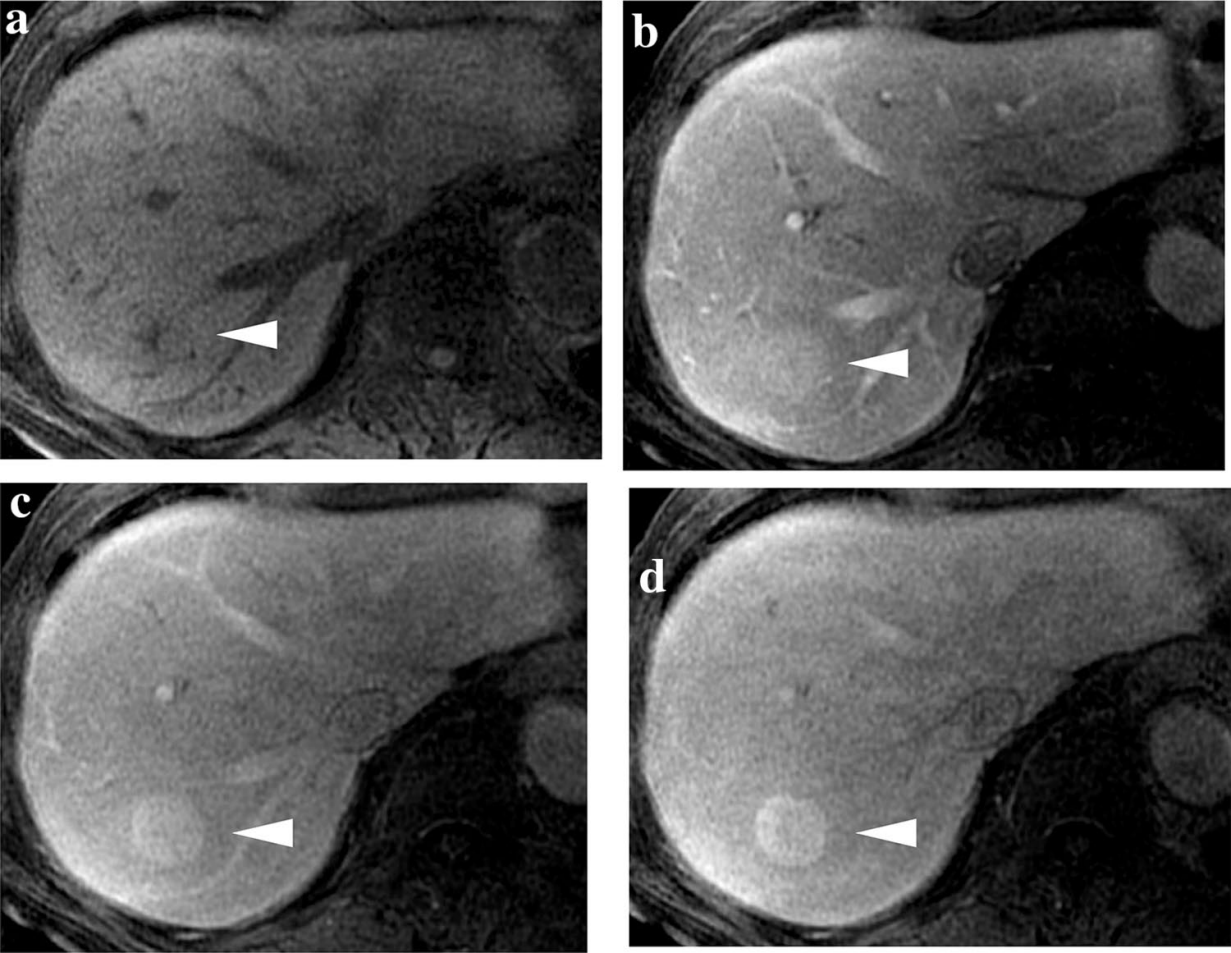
Images of multiple phase dynamic MRI on T1-weighted images for the nodule in segment 7 at 78 years of age. a On T1-weighted images, the lesion was hypointense (arrowhead). b–d The lesion was enhanced on dynamic MRI at 80, 120, and 180 s after contrast injection (arrowhead). *MRI* magnetic resonance imaging
